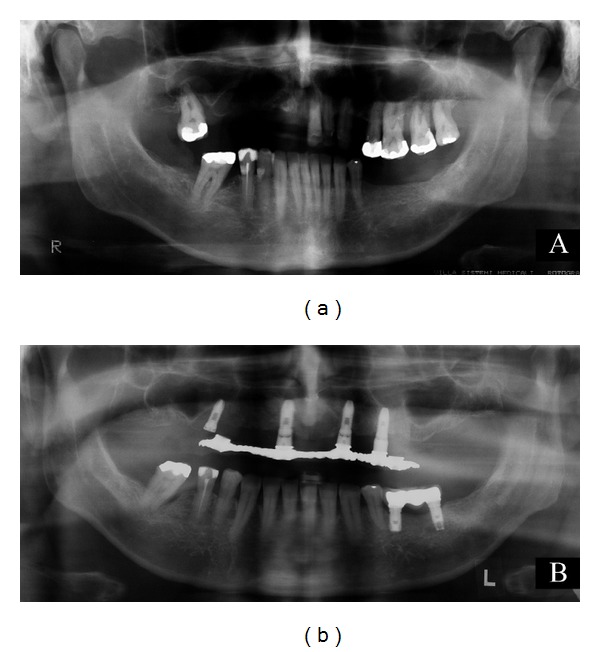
Panoramic radiographs of the patient. (a) Taken at the first visit. No bone alterations can be seen in the area of the lesion; (b) Nine-year follow-up: no change worthy of note.
